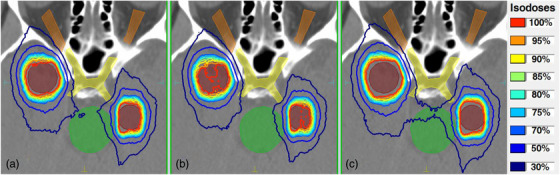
Axial CT slice with isodose lines (%) superimposed corresponding to (a) the reference plan, as well as plans with a systematic leaf positional offset of (b) −0.47 mm and (c) +0.47 mm, projected at the isocenter plane. Isolines are normalized to the prescription dose (i.e., 20 Gy). Contours legend: targets: maroon; brainstem: green; optic chiasm: yellow; optic nerves: brown
